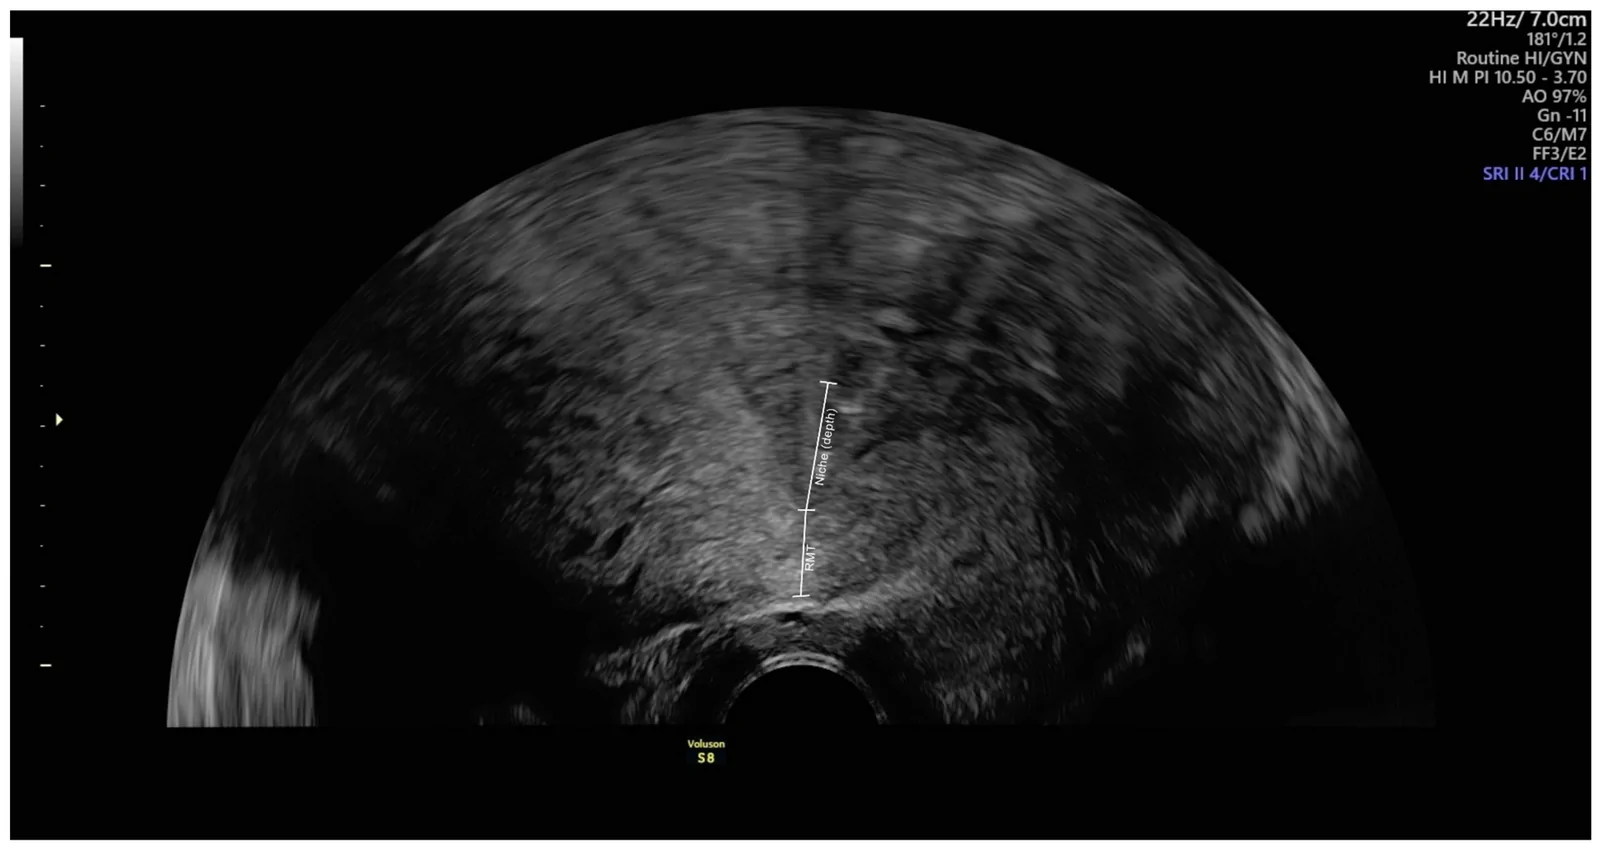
Uterus of a patient in sagittal section using transvaginal sonography (TVS). The lower uterine segment with a uterine defect after vaginal birth after caesarean section (VBAC) can be seen.
